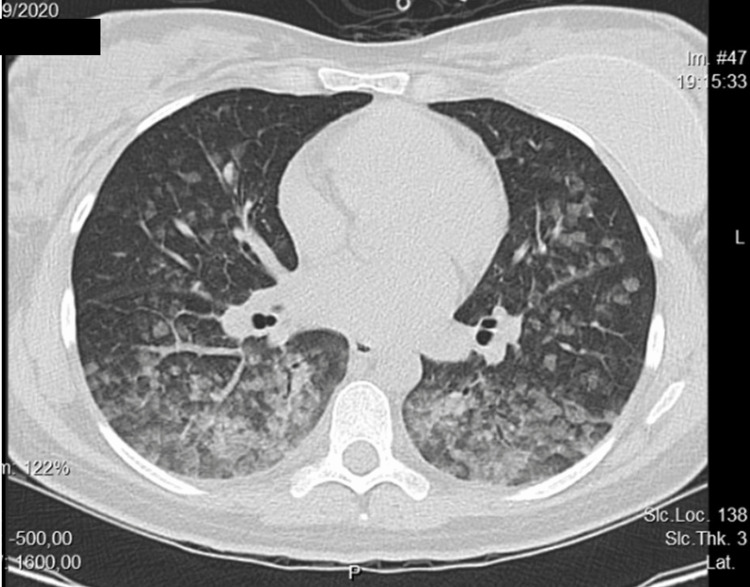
Thoracic CT scan, in a sagittal plane Bilateral pulmonary parenchymal infiltrations in a ground-glass pattern, more expressive on the upper segments of the inferior lobes, suggest bilateral hemorrhagic infiltration. A thin layer of bilateral pleural effusion is also seen.
